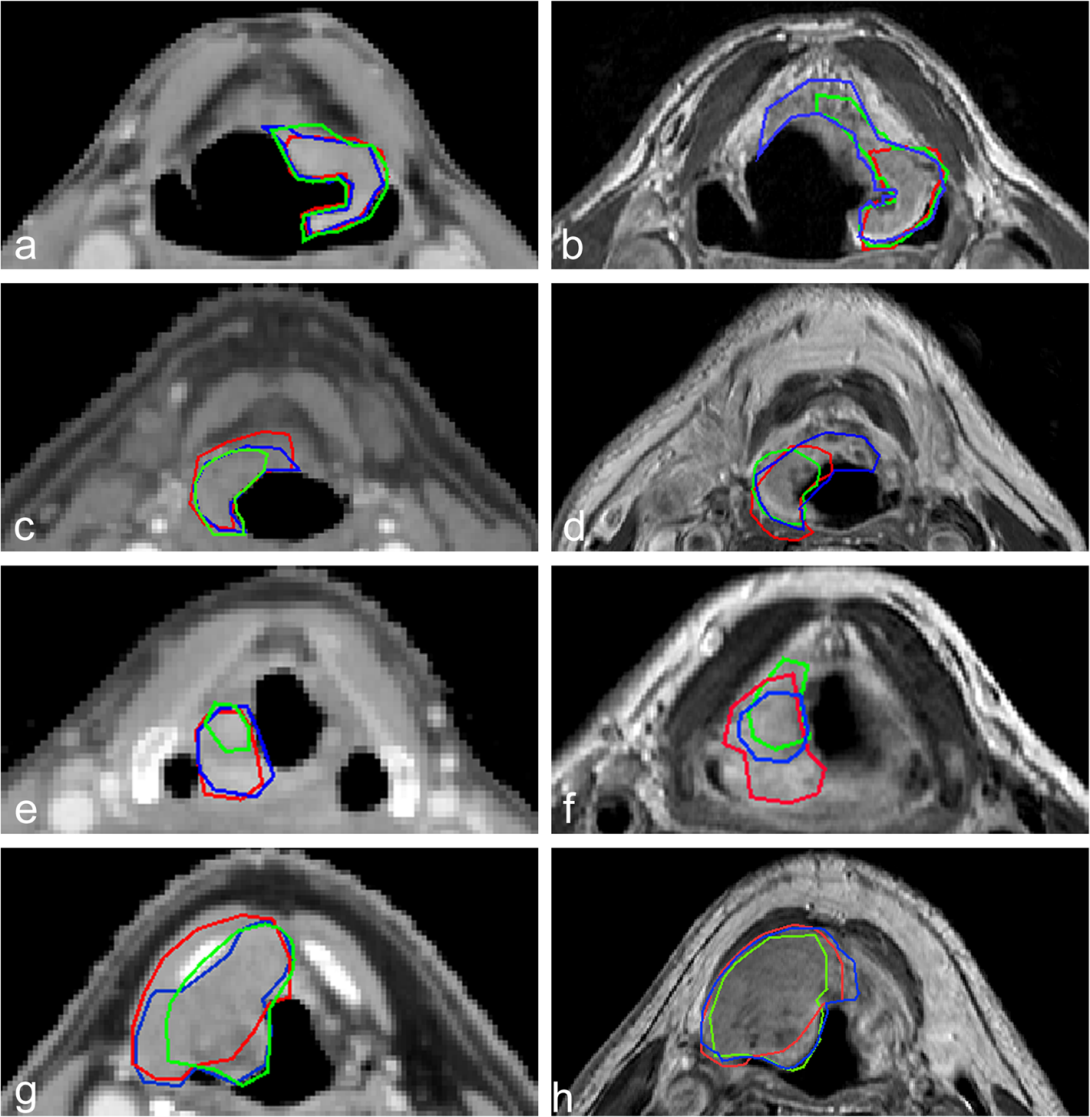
Delineations of three observers on contrast enhanced CT and CT-MR. Delineations of three observers on contrast enhanced CT (a, c, e, g) and on CT-MR (b, d, f, h) (T1-weighted + Gd) for four different patients.
